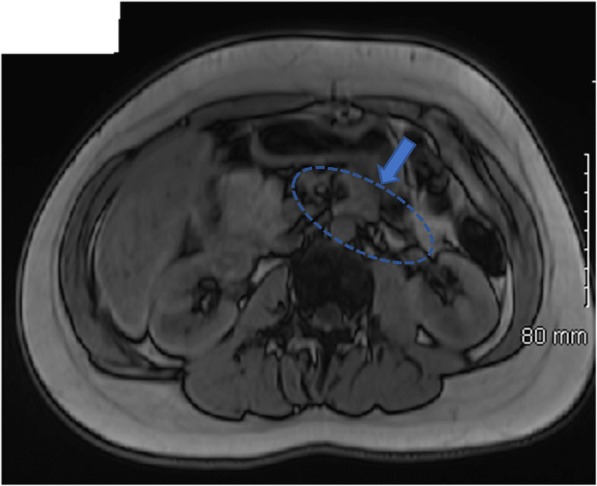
Abdominal MRI axial and coronal image revealing the absence of most of the neck, body and tail of pancreas
